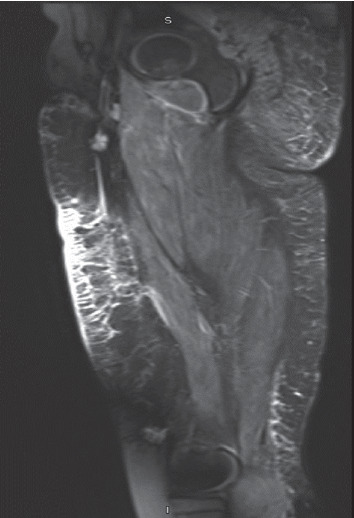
MRI imaging of thigh. Sagittal fat suppressed T2-weighted images revealing muscle edema and calcifications in the fascial planes.
